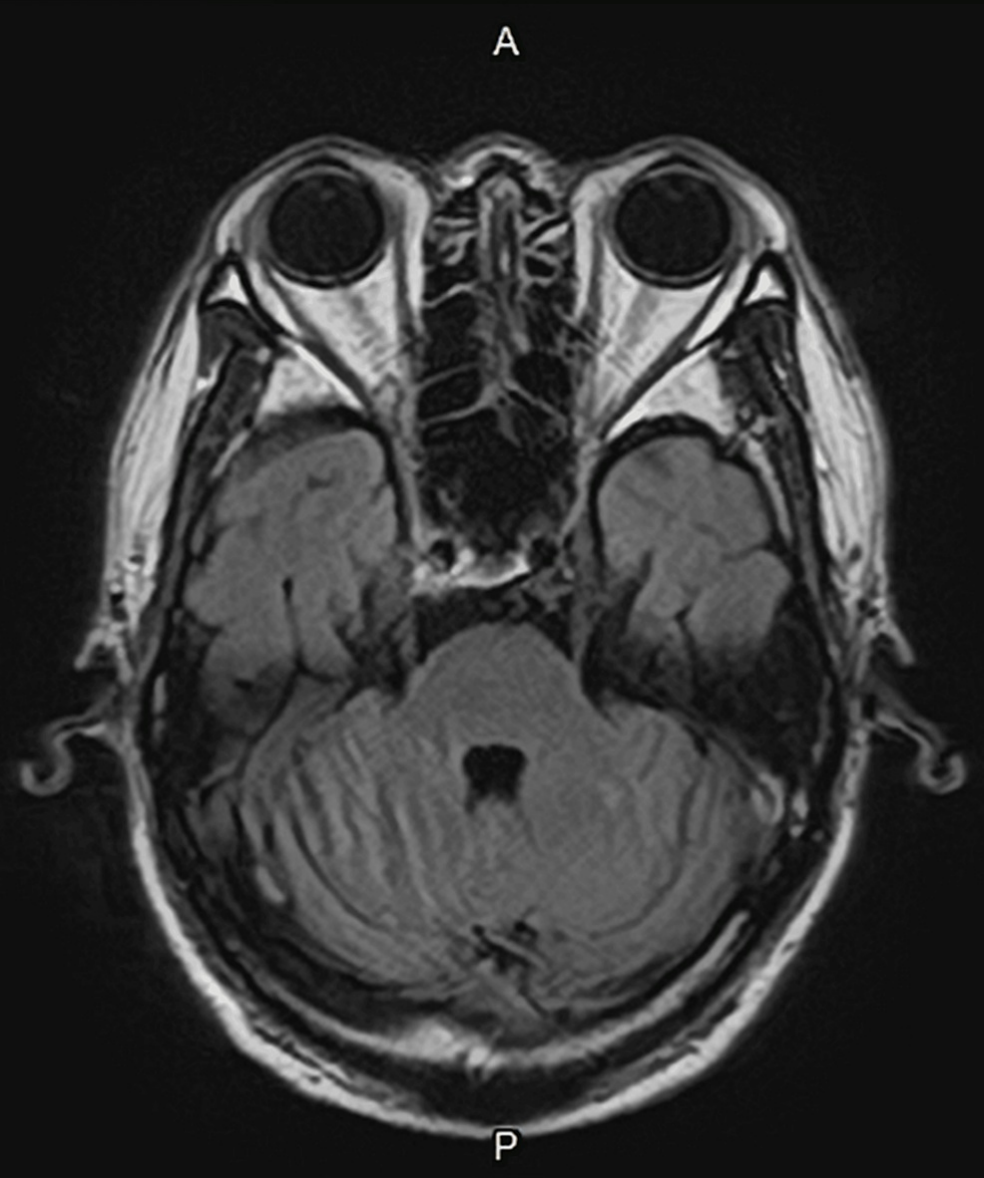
Contrast-enhanced magnetic resonance imaging of the brain and orbits. T2-weighted-fluid-attenuated inversion recovery revealed no inflammation or occupying lesion along the visual pathway. A = anterior; P = posterior
